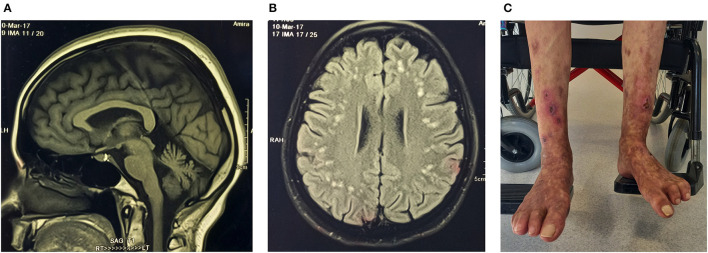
Proband (ICGNMD_8) with a milder phenotype of RFT1-congenital disorder of glycosylation. Brain imaging at age 35 years. (A) Sagittal T1-weighted MRI-brain scan showing cerebellar atrophy. (B) An axial brain FLAIR MRI image showing widespread discrete high signal intensity lesions in the subcortical white matter. Apart from the RFT1-disorders known to have coagulation factor abnormalities, the patient had no other cardiovascular risk factors to account for these white matter abnormalities of presumed vascular origin. (C) Spontaneous painful lesions with subsequent ulceration, which occurred in the distal extremities (before anticoagulation). Since oral anticoagulants were started for possible clotting abnormalities as a cause, the lesions have disappeared in the forearms and are substantially less in the legs (age 49).
